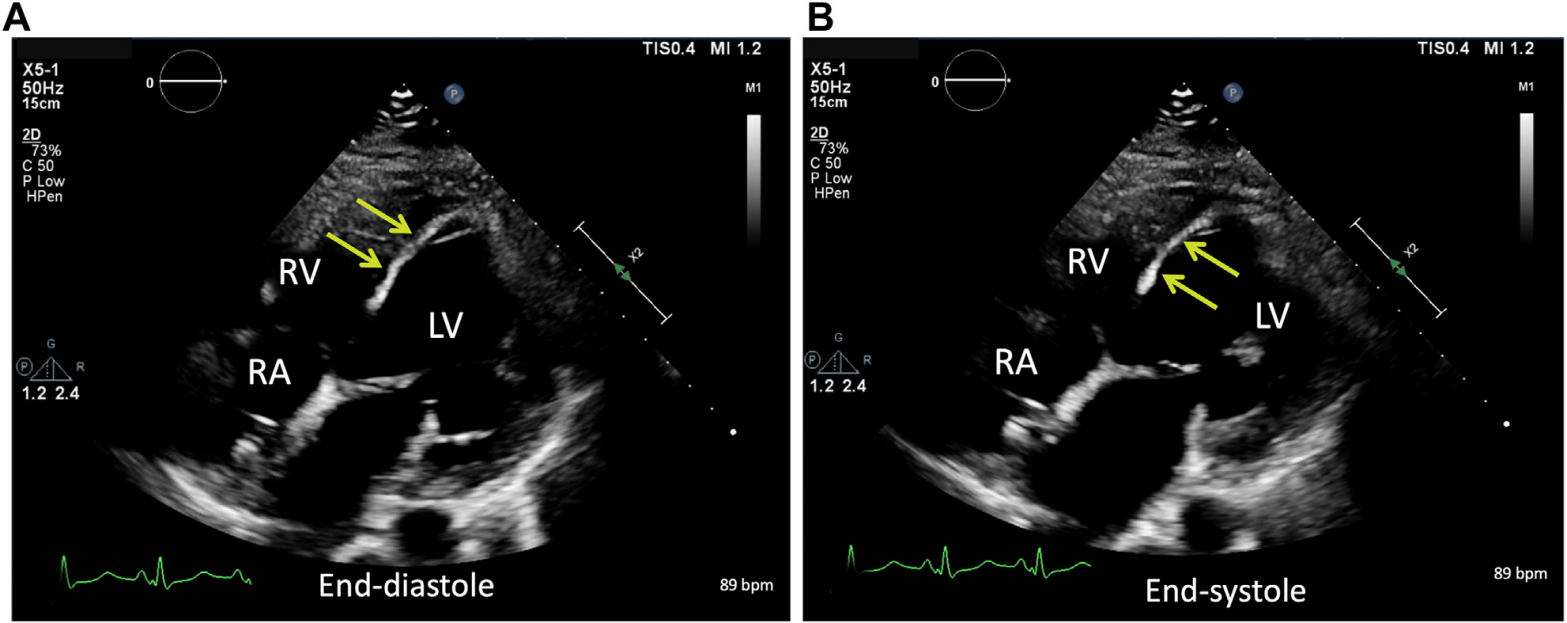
Two-dimensional TTE, right heart–focused apical four-chamber diastolic (A) and systolic (B) views at admission, demonstrates a relatively small RV compared with the left ventricular size, with the near midline IVS position becoming more rightward at end-systole. *LV*, Left ventricle; *RA*, right atrium.
